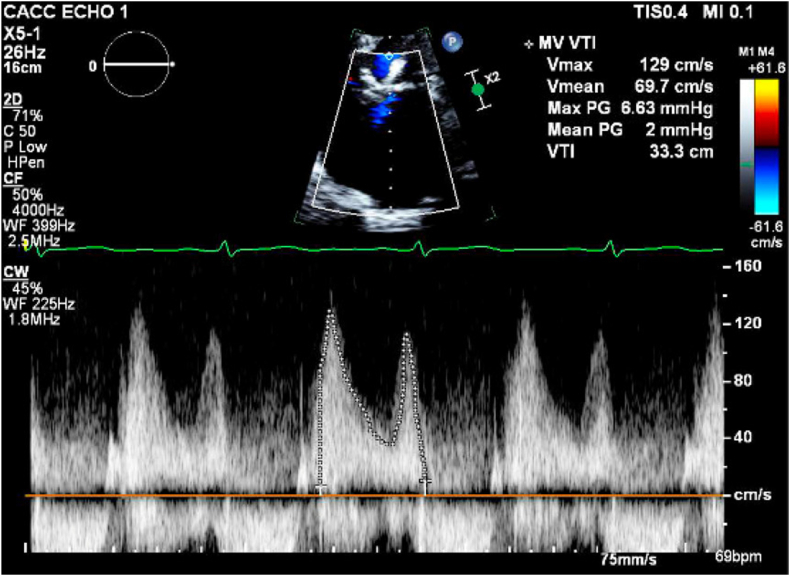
Three-month TTE showing mitral mean gradient of 2 mmHg by CW Doppler. Abbreviations: CF, frequency; CW, continuous wave; MI, magnification; MV, mitral vave; PG, peak gradient; TTE, transesophageal echocardiography; VTI, velocity time integral.
